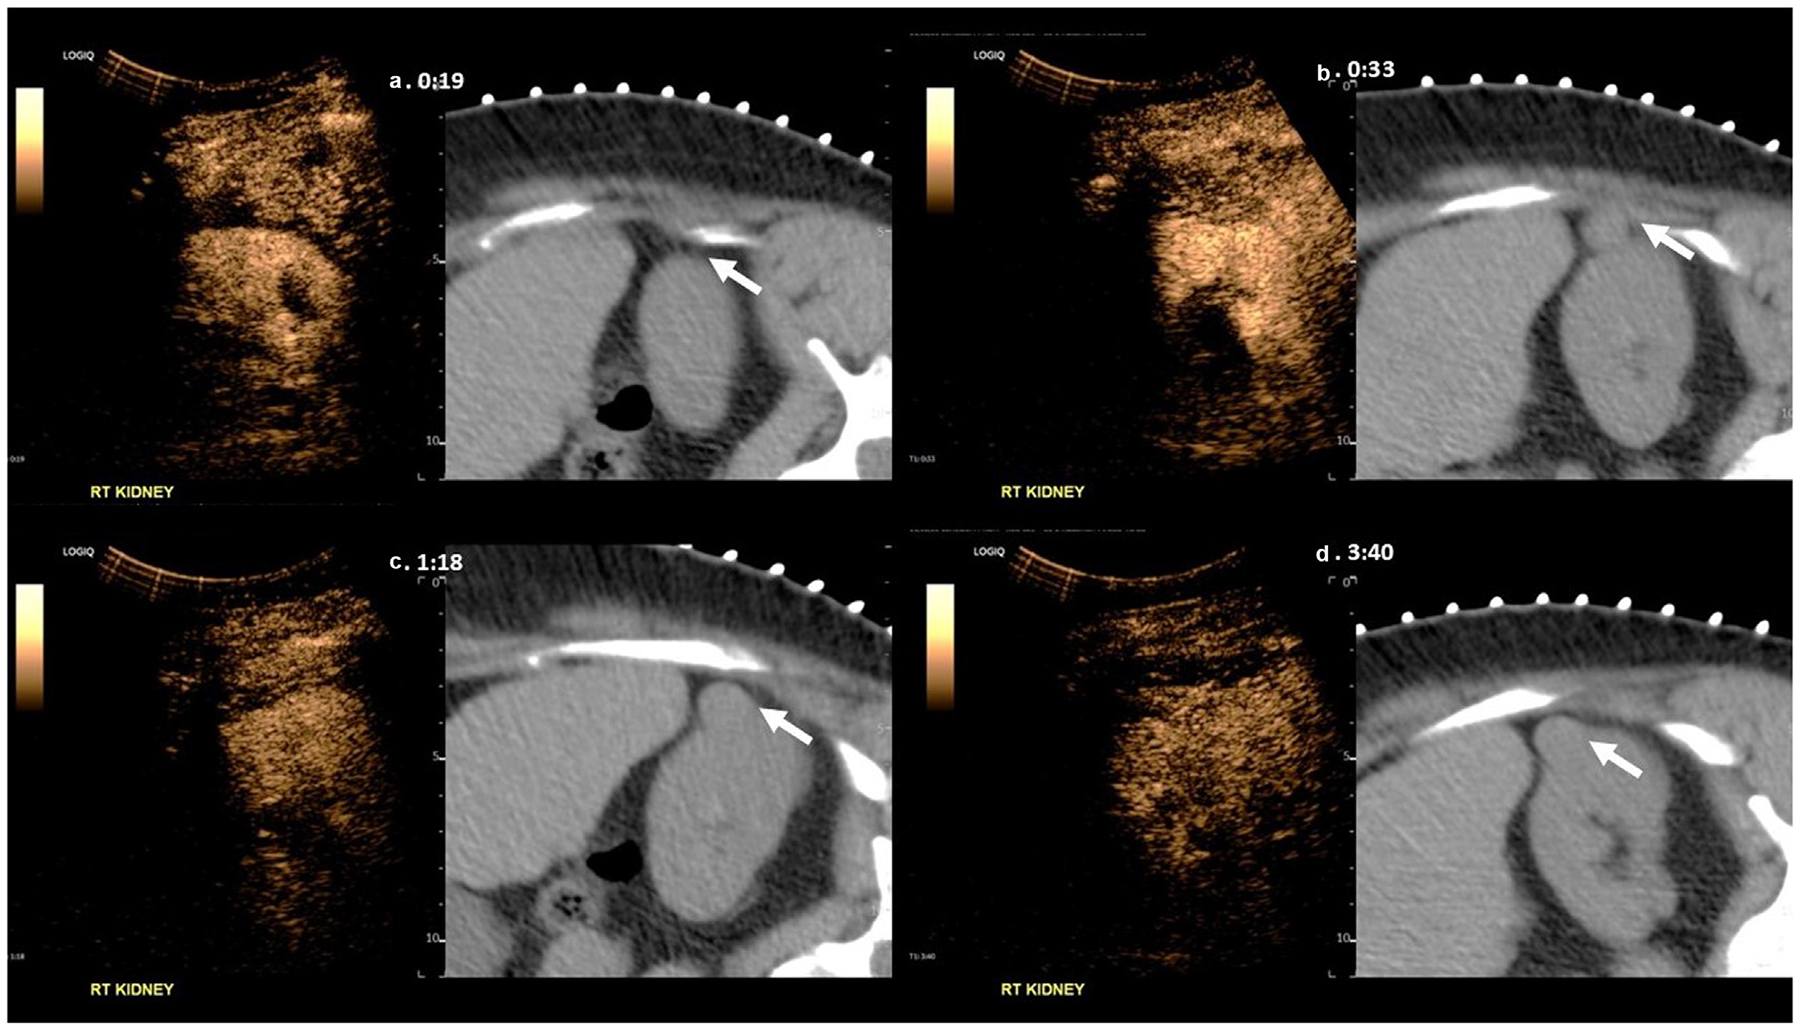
An example of a challenging case in which the region of interest on CEUS did not correctly match the patient’s preablation CT for fusion imaging. Note, that while the tumor is visualized well on the pretreatment MRI, the ablation cavity is not well appreciated on CEUS.
